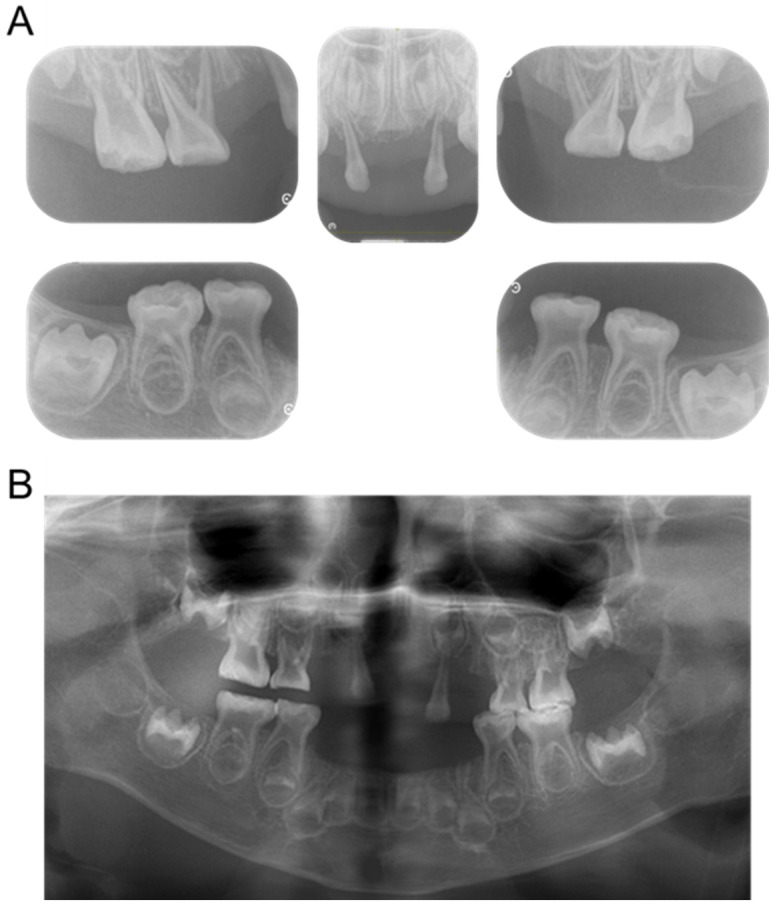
Radiographs taken at the age of 4 years and 4 months: periapical radiographs (A) and panoramic radiographs (B).
